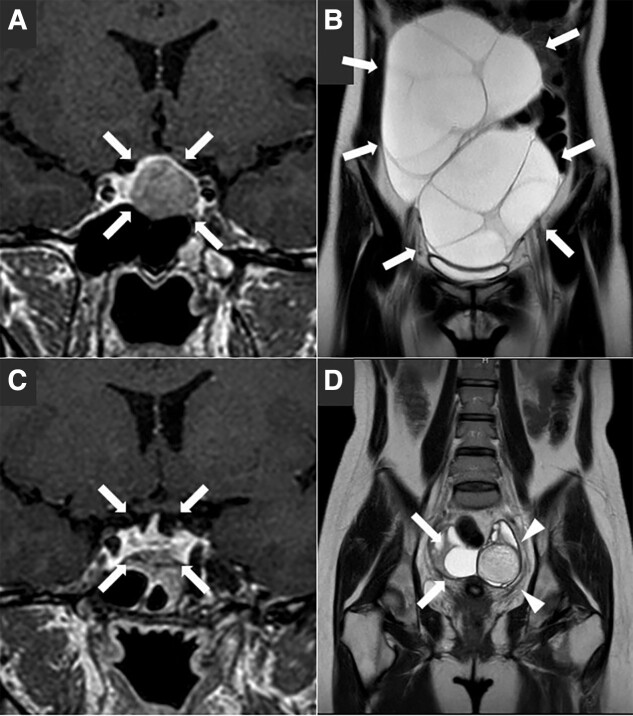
Coronal sections of pituitary or pelvic magnetic resonance imaging. A, A pituitary tumor with a maximum dimension of 19 mm (arrows) before surgery. B, Markedly enlarged multiple ovarian cysts of 15 cm (arrows) at presentation. C, Successful resection of the pituitary tumor (arrows) four months after surgery. D, Noticeably reduced ovarian cysts (arrows) and a 5 cm nodule suspected of a mature cystic teratoma (arrowheads) one month after the pituitary tumor resection.
